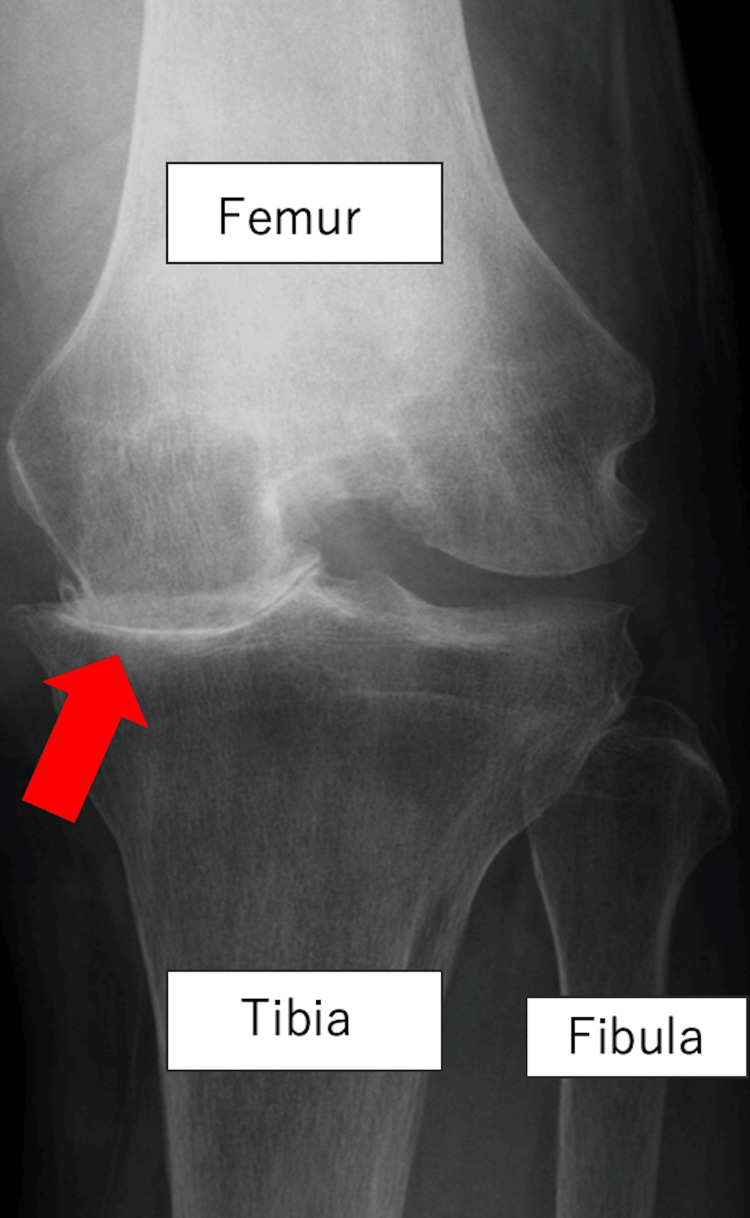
Preoperative X-ray shows the loss of the medial joint space (arrow).
